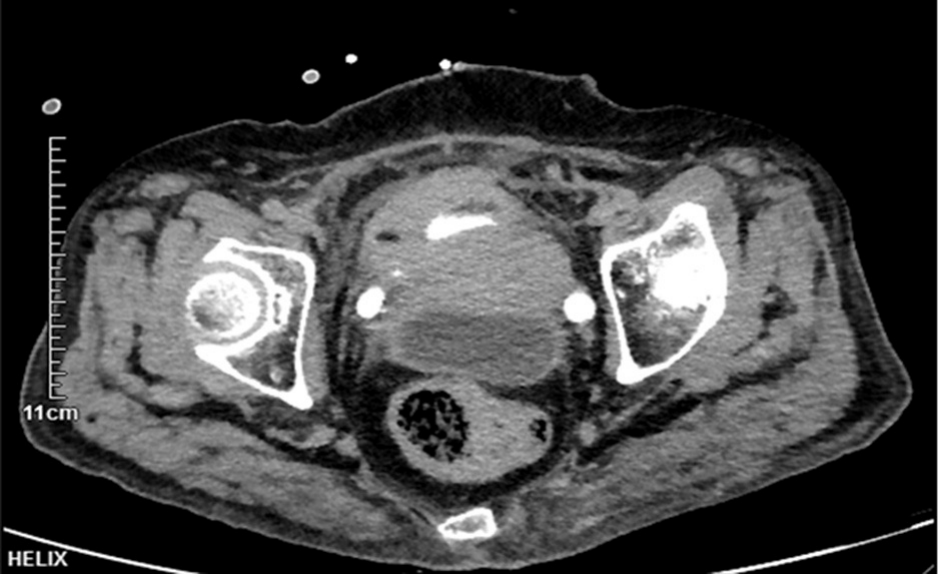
Coronal section CT urography of case 2. The bladder was filled with a contrast agent. The wall of the bladder was thickened. The CT scan demonstrated free fluid and thick-wall cysts in the pelvic cavity behind the uterus.
